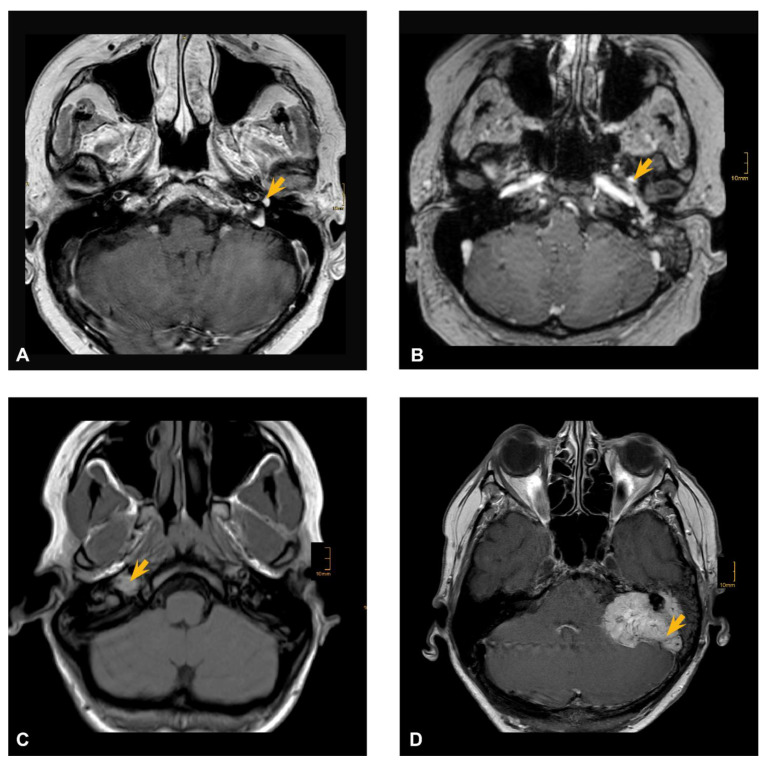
MRI examples of Fisch grades (A–D) in the respective population. (A) cranial MRI scan of Fisch A1 TBP, (B) cranial MRI scan of Fisch B3, (C) cranial MRI scan of Fisch C1, (D) cranial MRI scan of Fisch De2. The yellow arrow indicates tumor location in each radiological scan.
